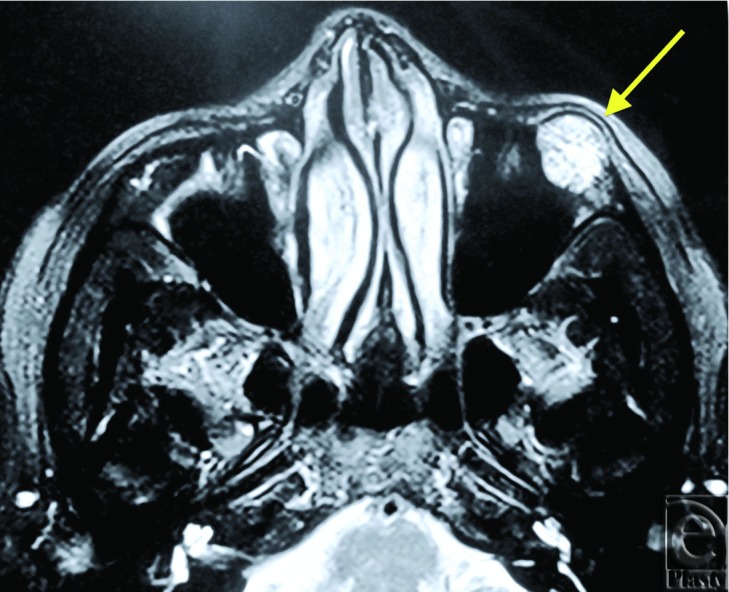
Axial MRI image of the infraorbital lesion.
